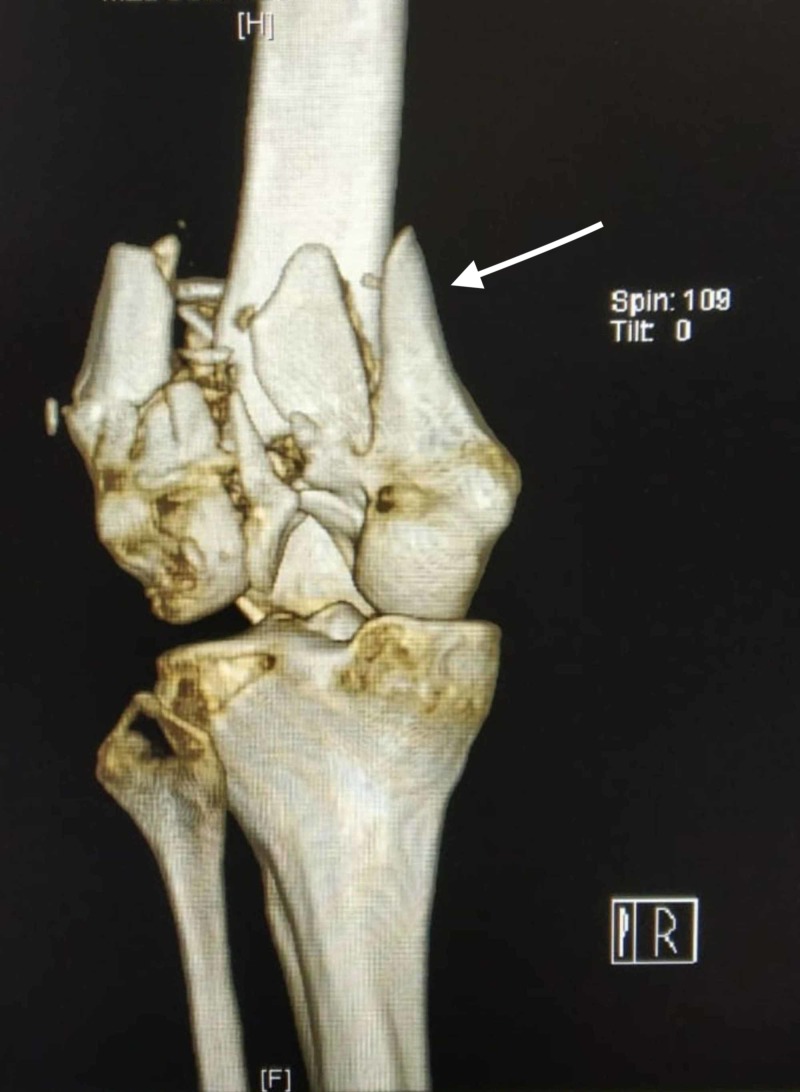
3D CT image showing a comminuted distal femur fracture in a 35-year-old male patient.
